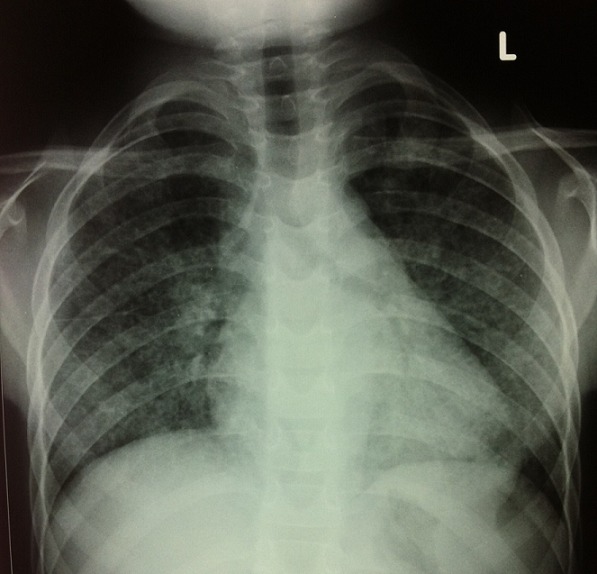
Postero-anterior chest X-rays showing bilateral diffuse nodular infiltrates, similar to miliary TB
